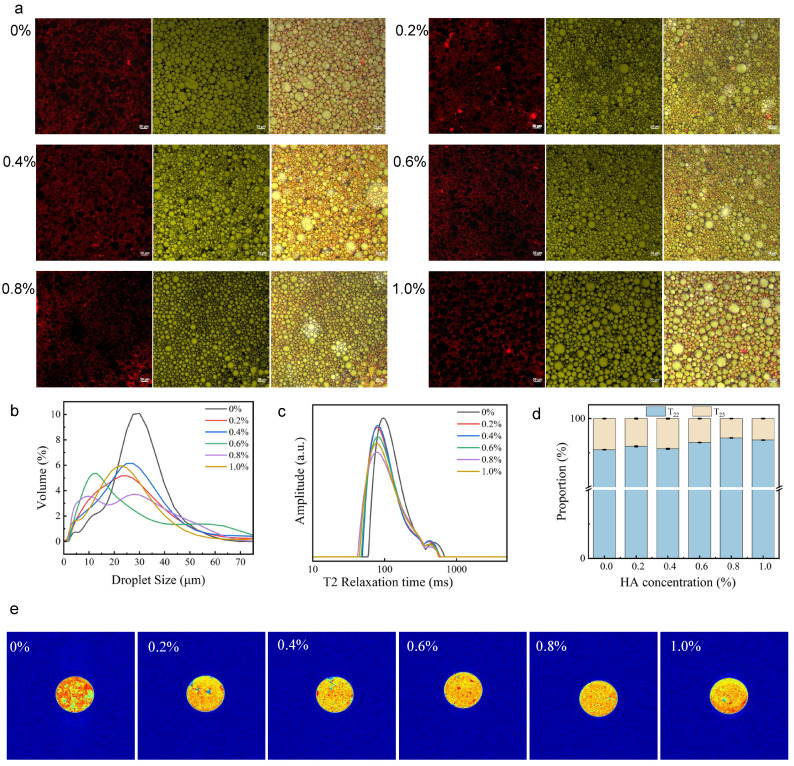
(a) CLSM images of HIPGEs (scale: 20 μm), (b) particle size distribution of HIPGEs, (c) T_2_ relaxation curve of HIPGEs, (d) T_22_ and T_23_ Relaxation Percentages of HIPGEs, (e) nuclear magnetic resonance imaging of HIPGEs.
